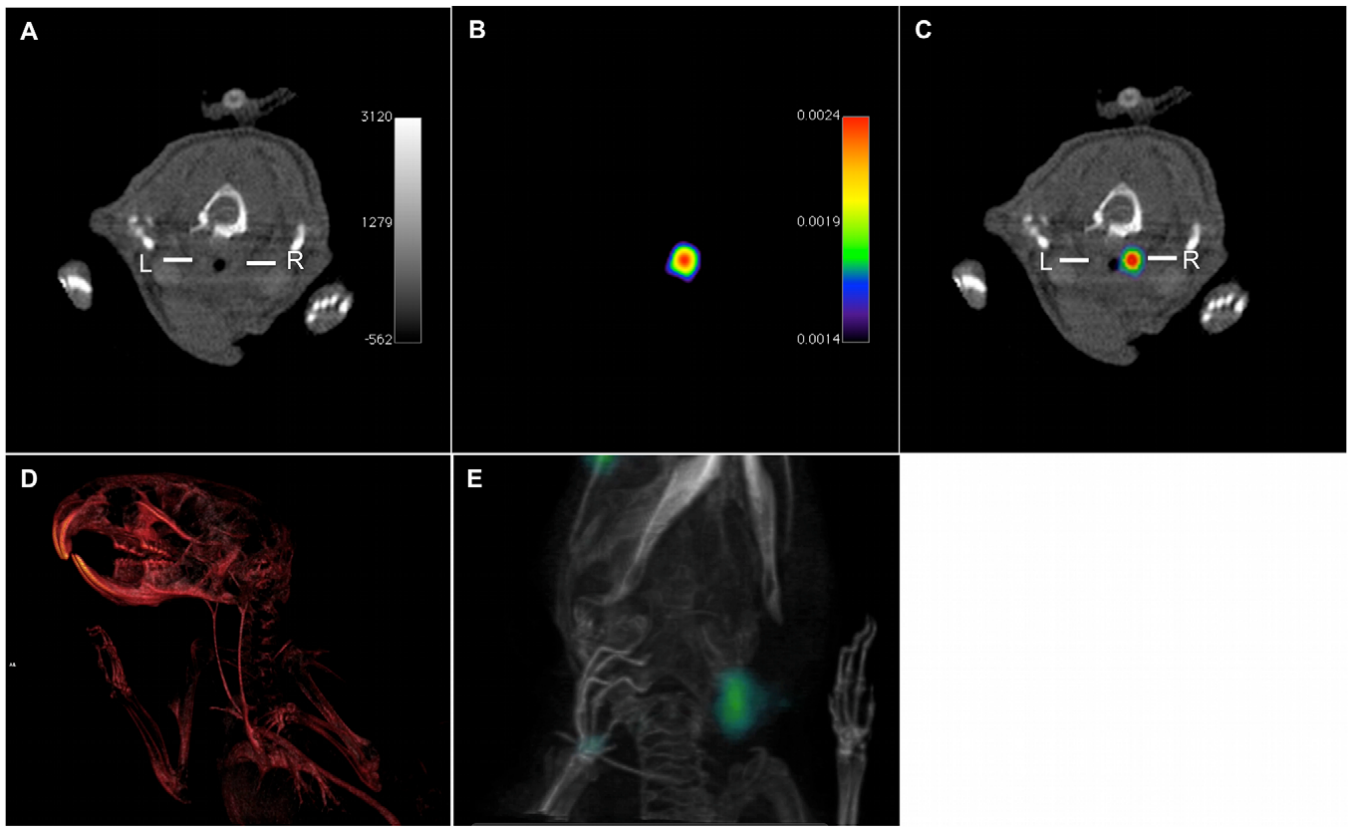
In vivo SPECT-CT imaging of carotid artery thrombosis. *In vivo* SPECT-CT single (A, B) and fused (C) images
